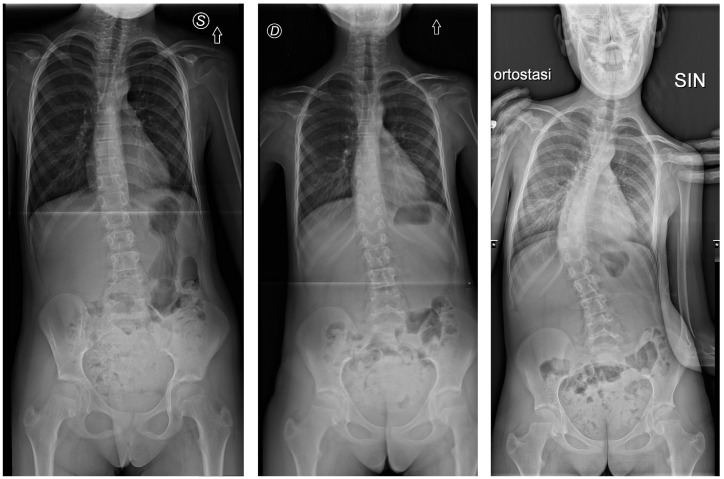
From (left) to (right), radiographs of participants no. 10 were collected at T0, T1, and T2, respectively. Letters “D” explicitates the right side of the body. The letter “S” and “SIN” indicate the left side of the body. The arrows indicate the upper margin of the radiographs.
